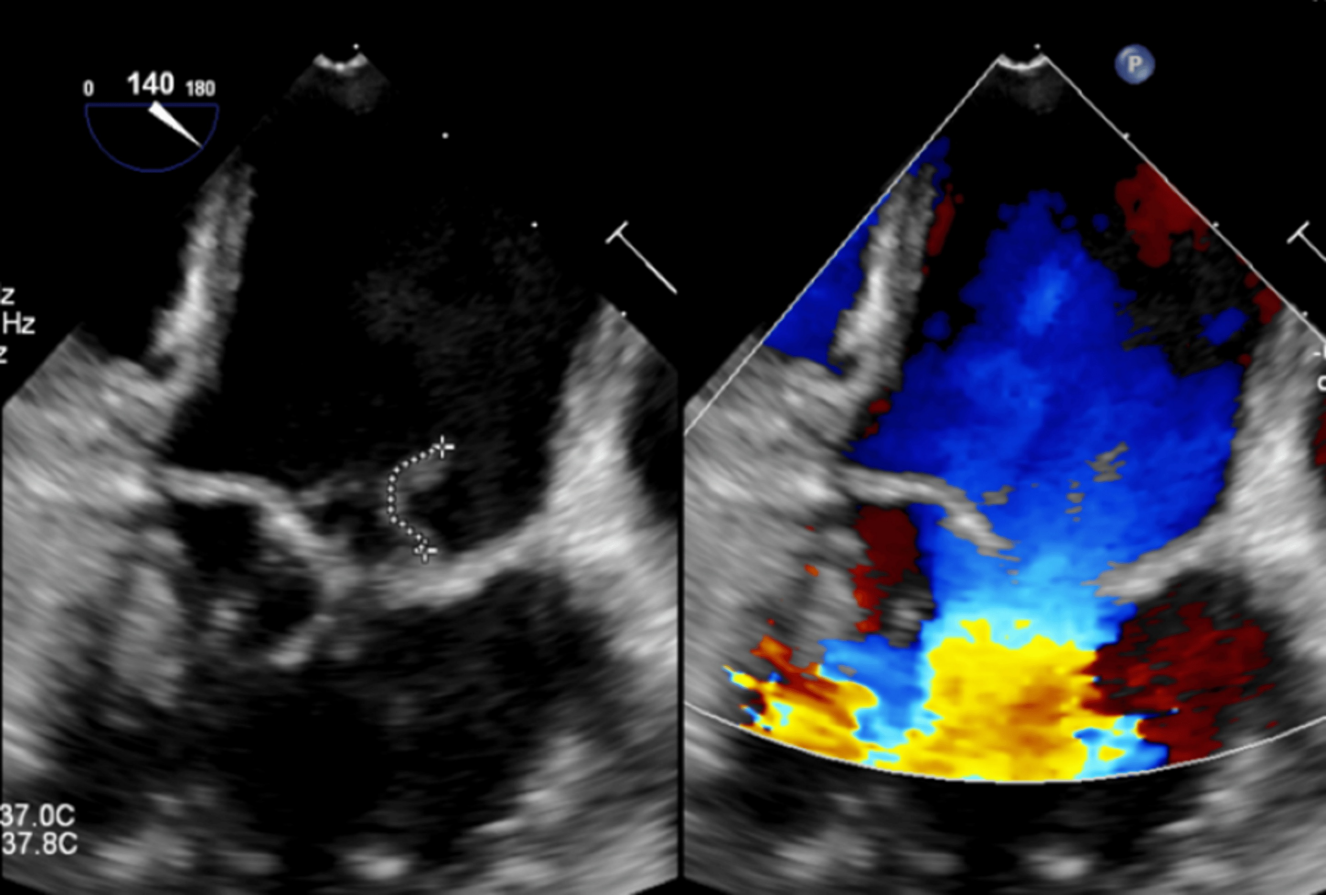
Transesophageal echocardiography showing mitral valve vegetations Transesophageal echocardiography revealed new-onset severe mitral regurgitation that was not observed on preoperative imaging. Two mobile, filamentous echo densities measuring 7.4 mm and 11.6 mm were visualized on the A2 segment of the anterior mitral valve leaflet, suggestive of vegetations.
